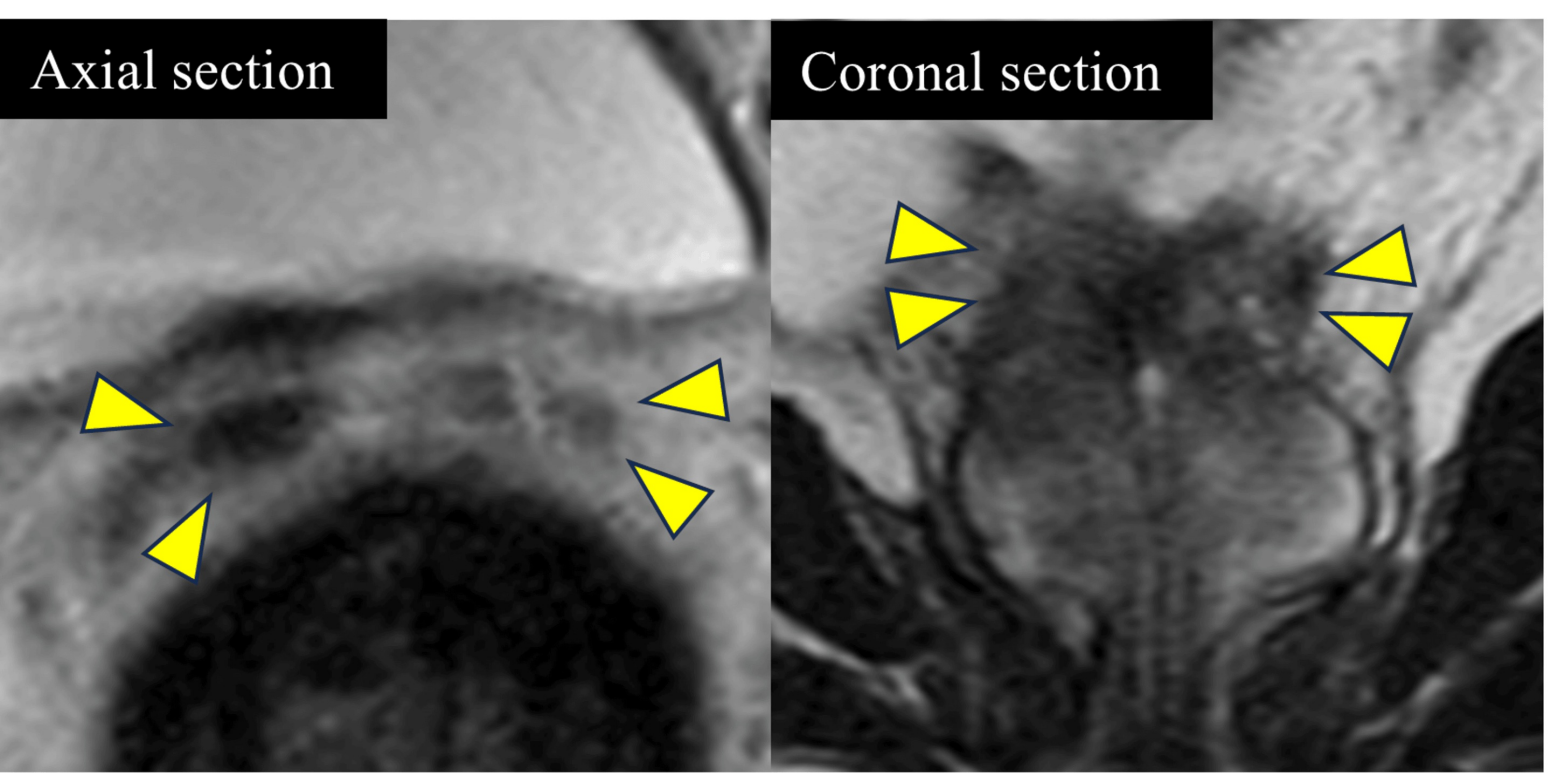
Atrophied seminal vesicles on MRI after conservative treatment No high-signal areas were observed within the bilateral seminal vesicles (indicated by arrowheads). The findings were suggestive of seminal vesicle atrophy and decreased seminal fluid.
